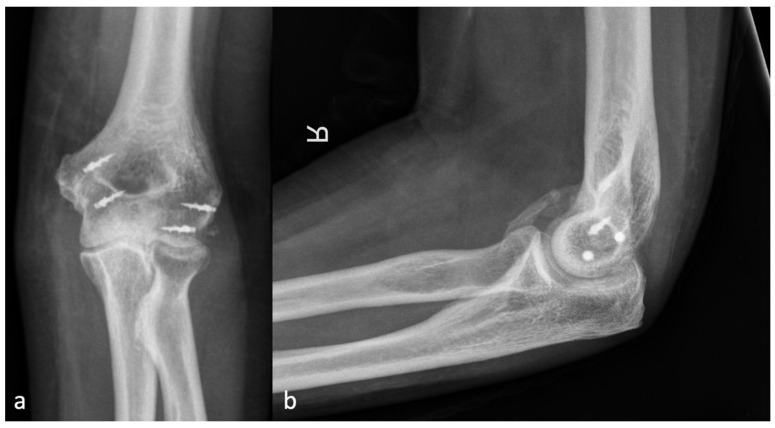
(a) shows the AP and (b) shows the lateral X-ray with an anterior HO forming a brace from the humerus to the forearm, representing an HO 3a.
